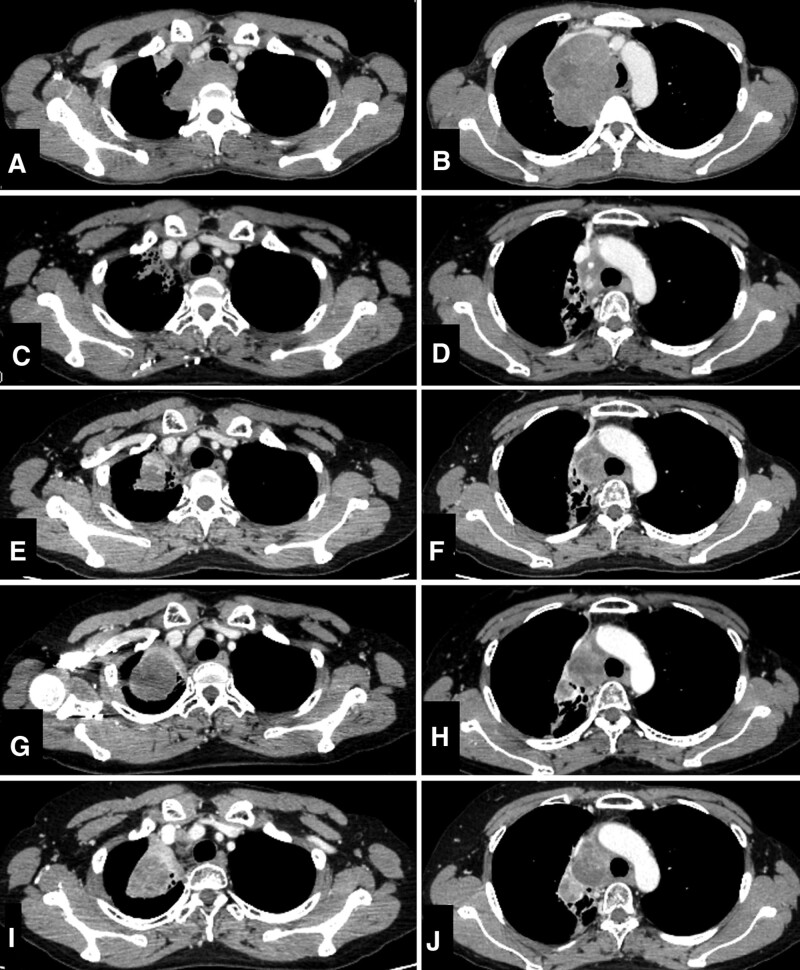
Contrast enhanced computed tomography of the tumor from pretreatment to third-line cytotoxic chemotherapy. When comparing the tumor images before chemotherapy (A, B) with those after 5 cycles of VDC/IE and radiotherapy (C, D), a significant reduction in tumor size was observed. However, following 2 cycles of VDC/IE, the tumor began to exhibit growth (E, F). Subsequently, after undergoing 2 cycles of topotecan and cyclophosphamide combination chemotherapy, the tumor growth persisted (G, H). The initiation of third-line chemotherapy consisting of 3 cycles of irinotecan and temozolomide led to stable disease, as indicated by the CT scans (I, J). CT = computed tomography, VDC/IE = Vincristine, doxorubicin, and cyclophosphamide, alternating with ifosfamide and etoposide.
